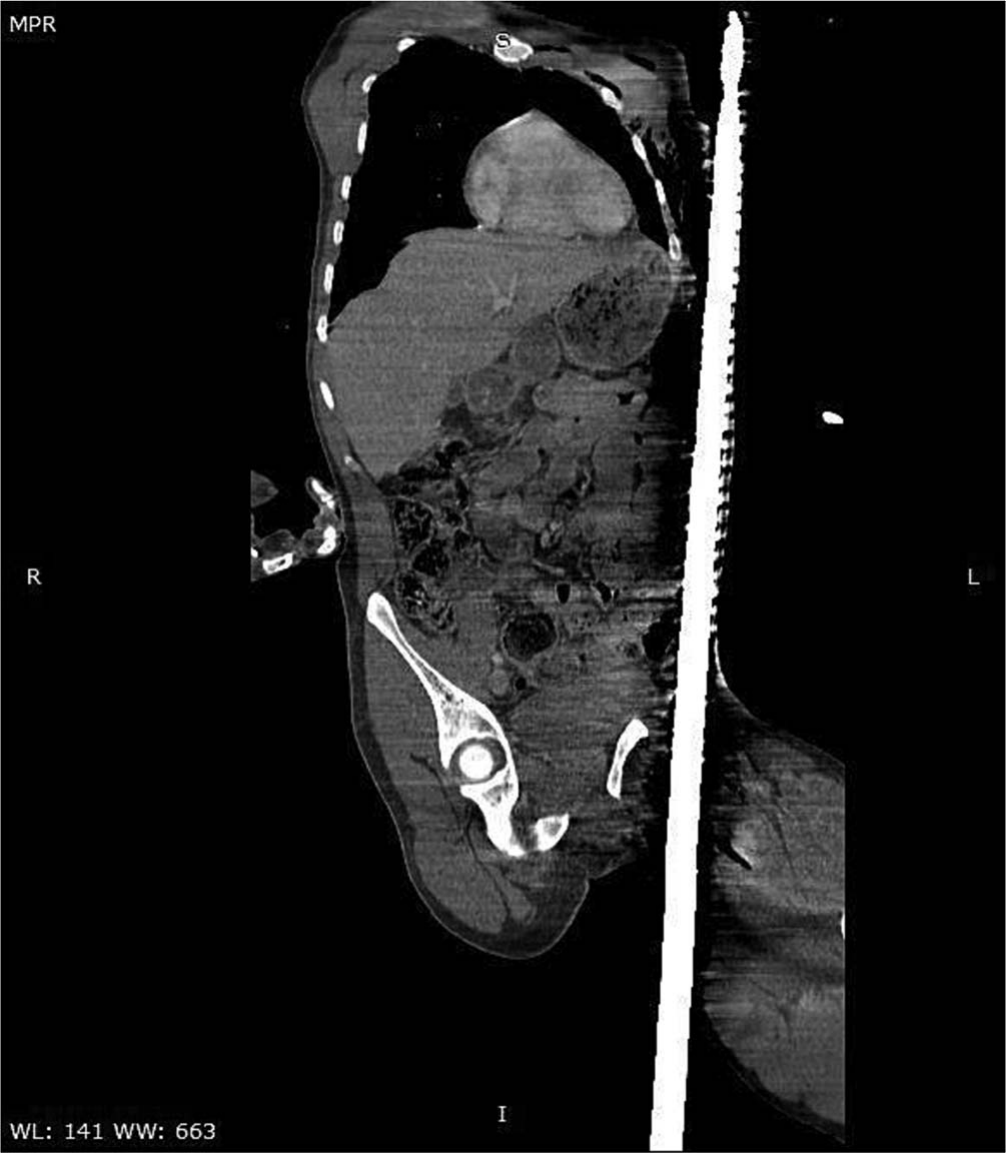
Sagittal CT image demonstrating the trajectory of the metallic rod traversing the body through the subcutaneous and epifascial planes.
